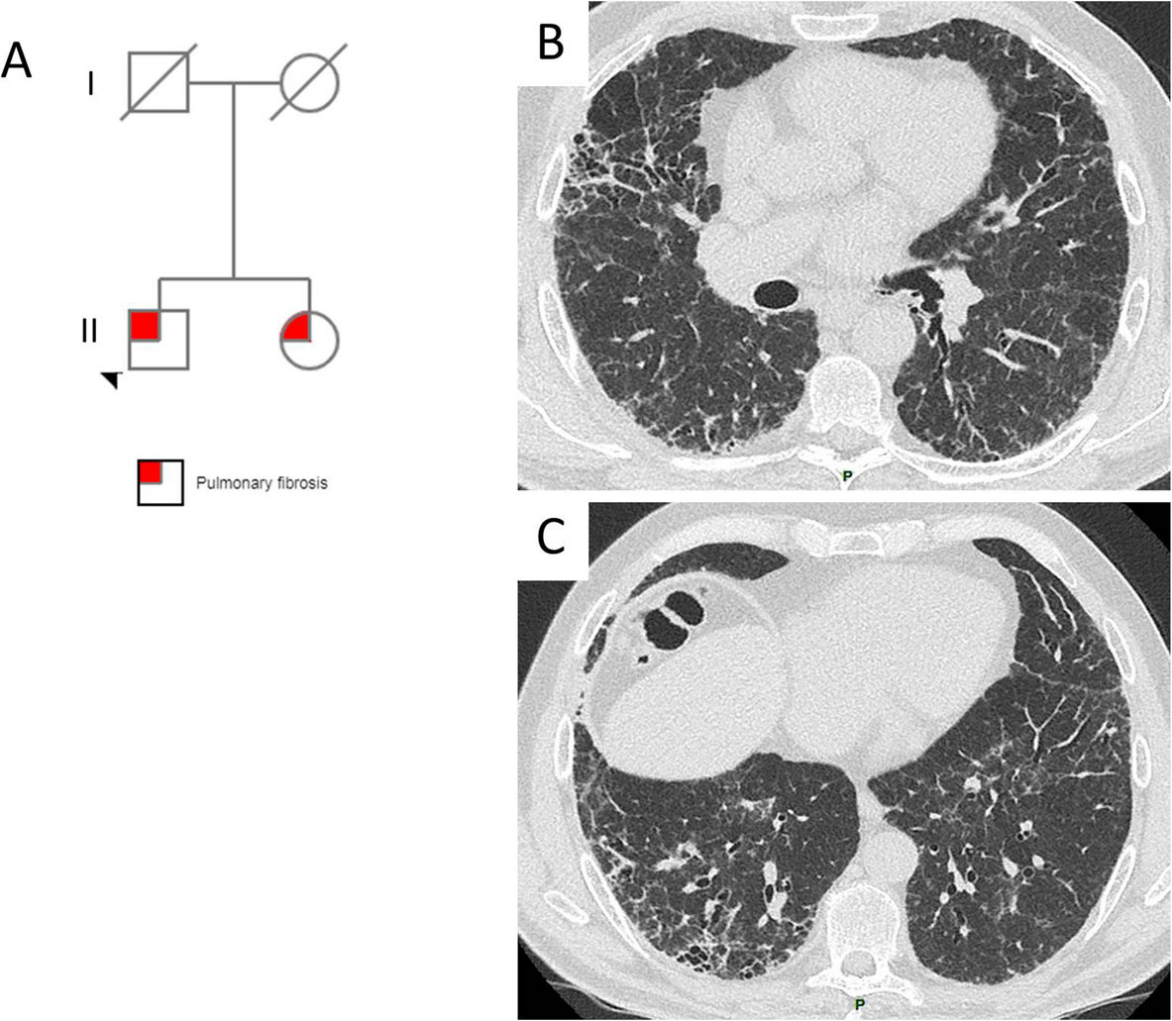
(a) A 64-year-old non-smoking man with familial pulmonary fibrosis and no extra-pulmonary manifestation. (b, c) The CT scan pattern was considered usual interstitial pneumonia (UIP). Genetic analysis revealed a heterogeneous *TERT* mutation (c.3216G > A, p.Trp1072*), classified as pathogenic, in both siblings. Genetic counselling was proposed for the relatives. Antifibrosing therapy was offered along with lung transplantation screening for the proband
